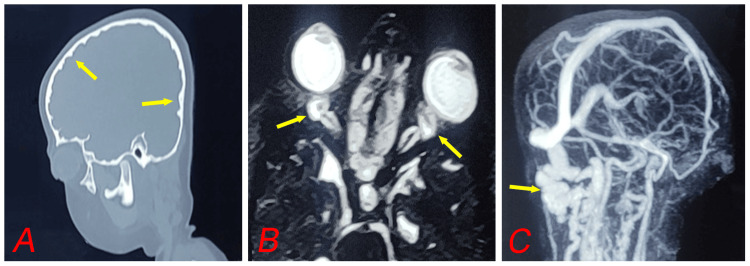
CT brain demonstrating a copper-beaten appearance of the skull, indicative of chronic raised intracranial pressure (ICP) with prominent impressions (arrows) of brain gyri on the inner table of the skull (A), T2-weighted gadolinium-enhanced MRI (CEMRI) of the brain and orbit showing severe optic nerve sheath oedema (arrows) (B), and Magnetic resonance venography (MRV) revealing collateral venous pathways and venous shunting (arrow), indicative of chronic intracranial hypertension and compensatory venous changes (C). CT: computed tomography; CEMRI: contrast enhanced magnetic resonance imaging.
